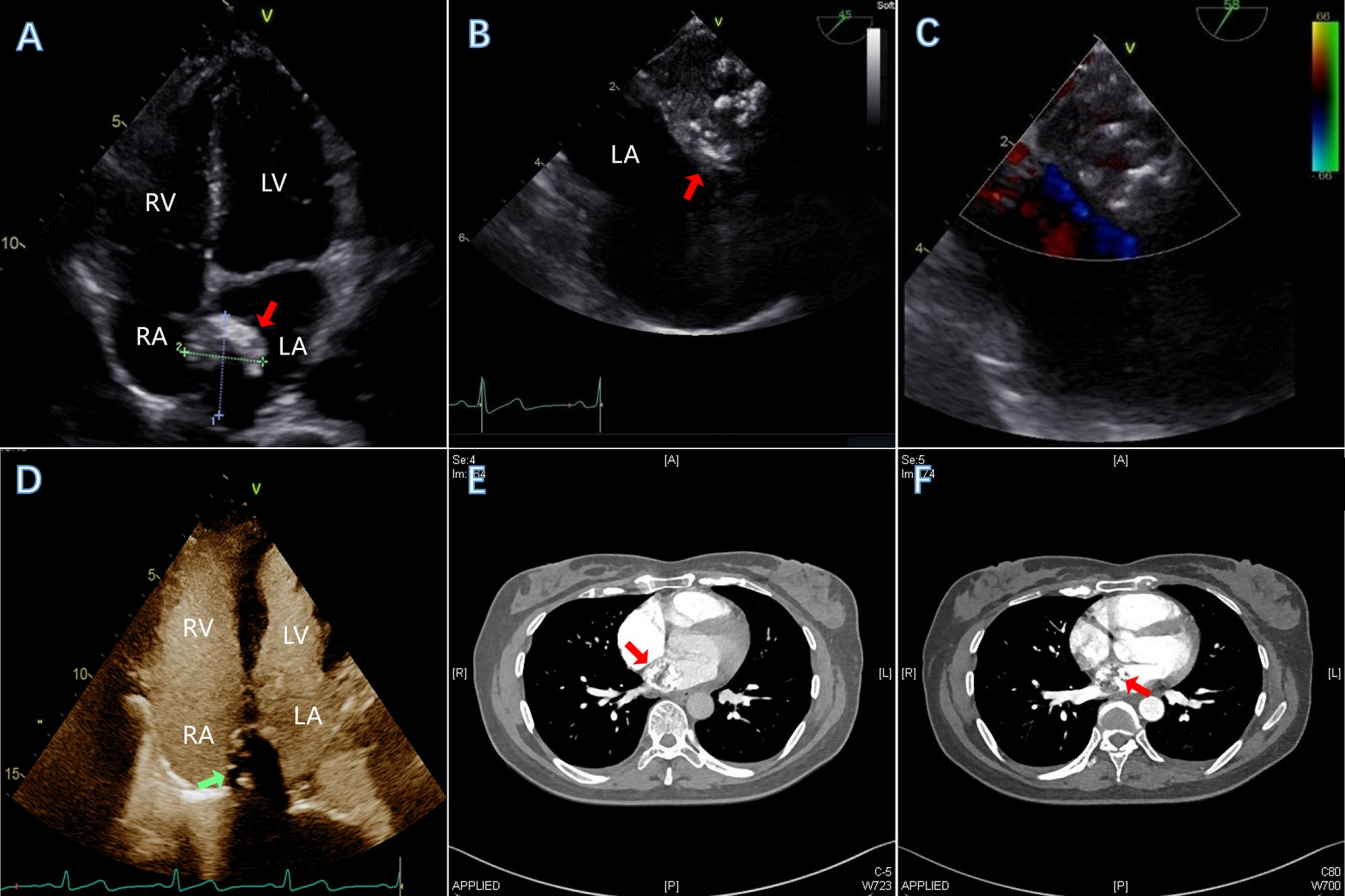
Multiparametric imaging. In the four-chamber view on TTE, a mass was detected in the left atrium, adjacent to the IAS. The mass was hyperechoic with significant posterior acoustic shadowing and exhibited minimal mobility, as indicated by the red arrow in image (A). TEE (two-dimensional and color Doppler ultrasound) showed left atrial mass(B and C). The cTTE revealed a small amount of contrast agent (green arrow) infiltrating the mass(D). CTA demonstrated that the mass in the left atrium was heterogeneously contrast enhanced during the venous phase and showed further enhancement during the arterial phase (E-F). *Note* LA, left atrium; LV, left ventricle; RA, right atrium; RV, right ventricle
